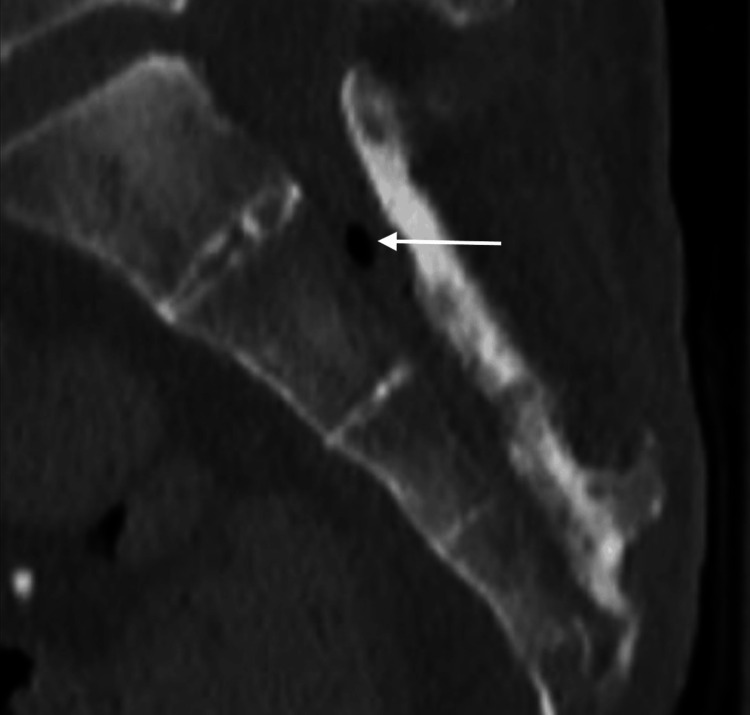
CT scan of sacrum and coccyx at additional sagittal plane Osteomyelitis of sacrum and coccyx with gas formation in spinal canal visualized on CT at different sagittal plane with evidence of ascending spinal canal infection (white arrow).
